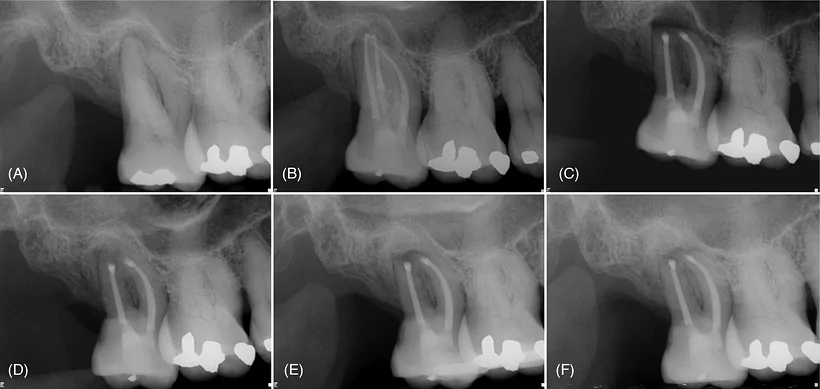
Case 1: Periapical radiographs of tooth #2 showing a radiolucent lesion surrounding the root (A) and post‐root canal treatment (B). Intraoperative radiograph following retropreparation and retrofilling of the buccal canals and complete palatal root resection (C). Immediate postoperative radiograph after guided bone regeneration (D). Radiographs at 1 month (E) and 14 months (F) demonstrating stable healing and bone fill.
